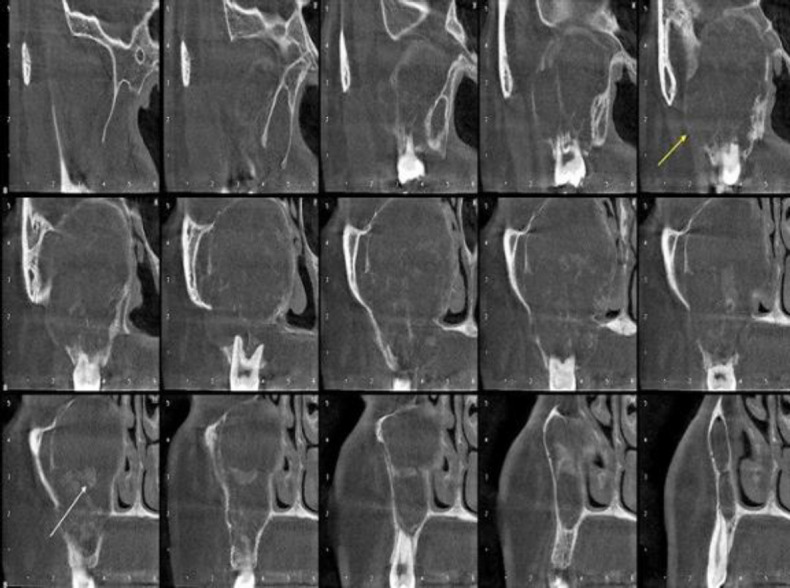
Coronal view of cone-beam computed tomography displayed an expansile lesion with ground glass pattern (short arrow) and erosion of the maxillary sinus’s wall (long arrow)
